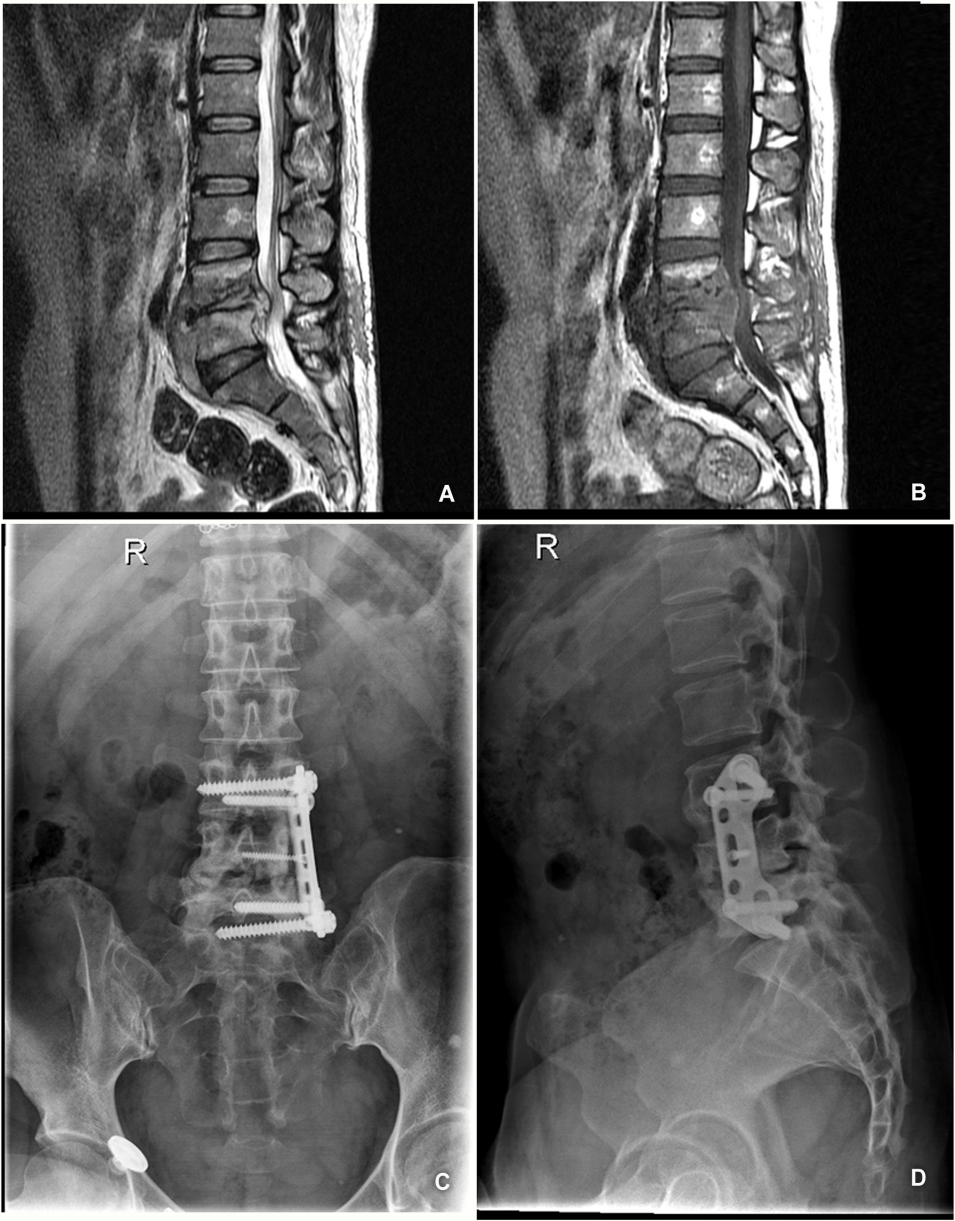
A 56-year-old woman with lumbosacral spinal tuberculosis received conservative anti-TB therapy. (A)Pretreatment MRI showed destruction of the L4–L5 vertebrae and formation of paravertebral abscess with concomitant compression of the spinal cord. (B) After 6-week anti-TB therapy, the back pain became more serious. (C,D) No recurrence of TB was noted in this patient during the follow-up period.
